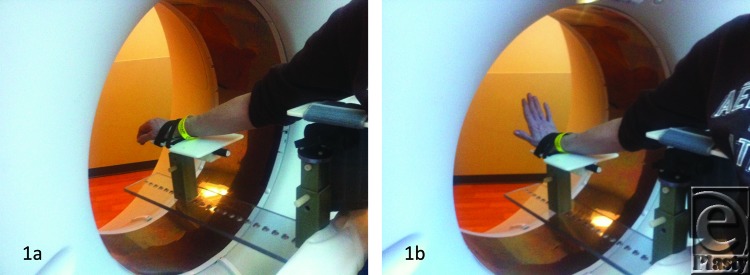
Dedicated “in CT” wrist platform. Pictures taken during unconstrained flexion and extension motions.
